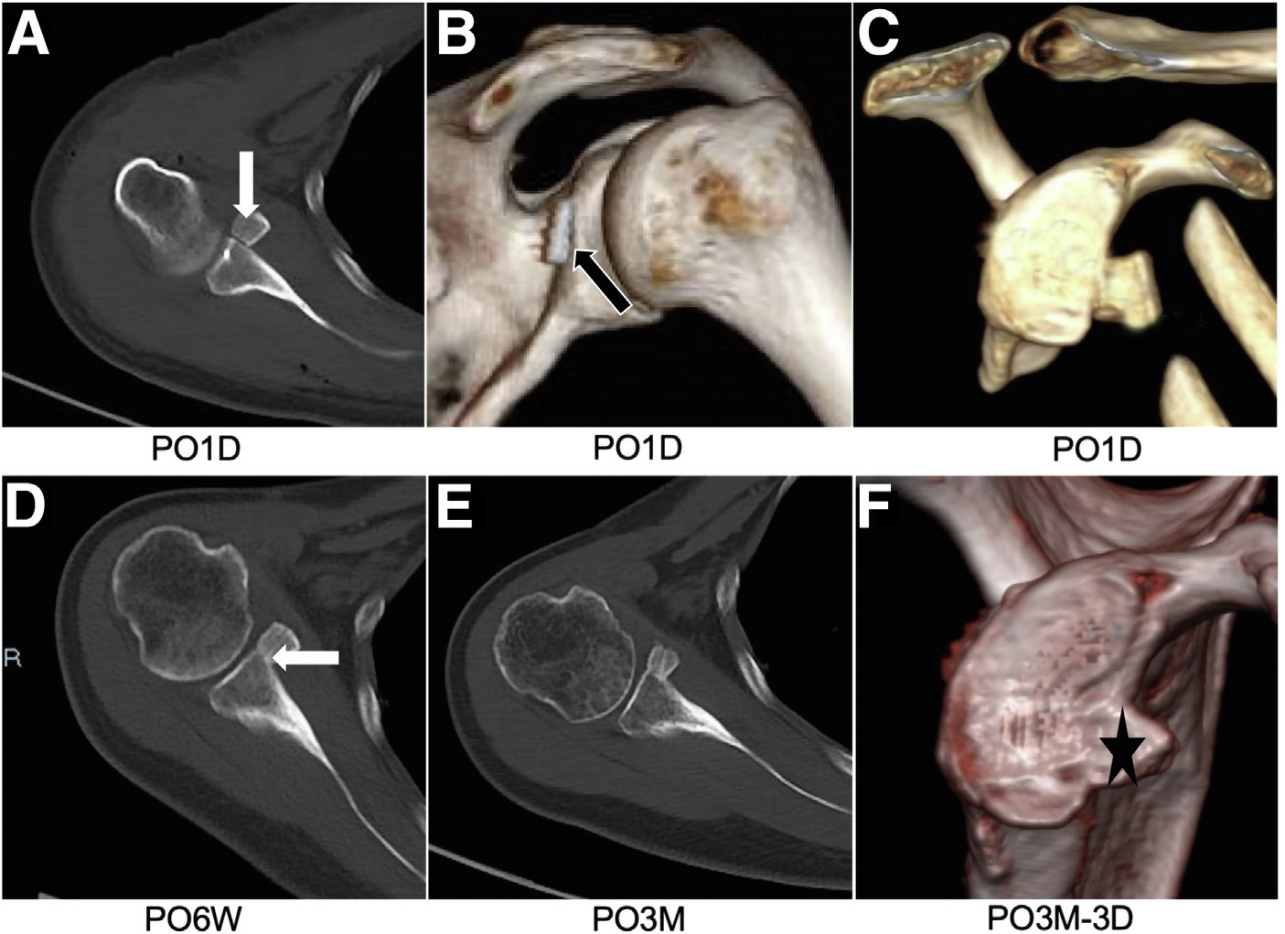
Computed tomography (CT) images of right (R) shoulders in typical patients at postoperative follow-up. (A-C) CT images on first day postoperatively (PO1D) showing good positioning of bone block (white arrow) and posterior EndoButton (black arrow). (D) CT scan at 6 weeks postoperatively (PO6W) showing good bone healing (white arrow). (E, F) Complete bone healing and remodeling of glenoid (black star) at 3 months postoperatively (PO3M). PO3M-3D, post-operatively 3 months-3 dimensional reconstruction.
